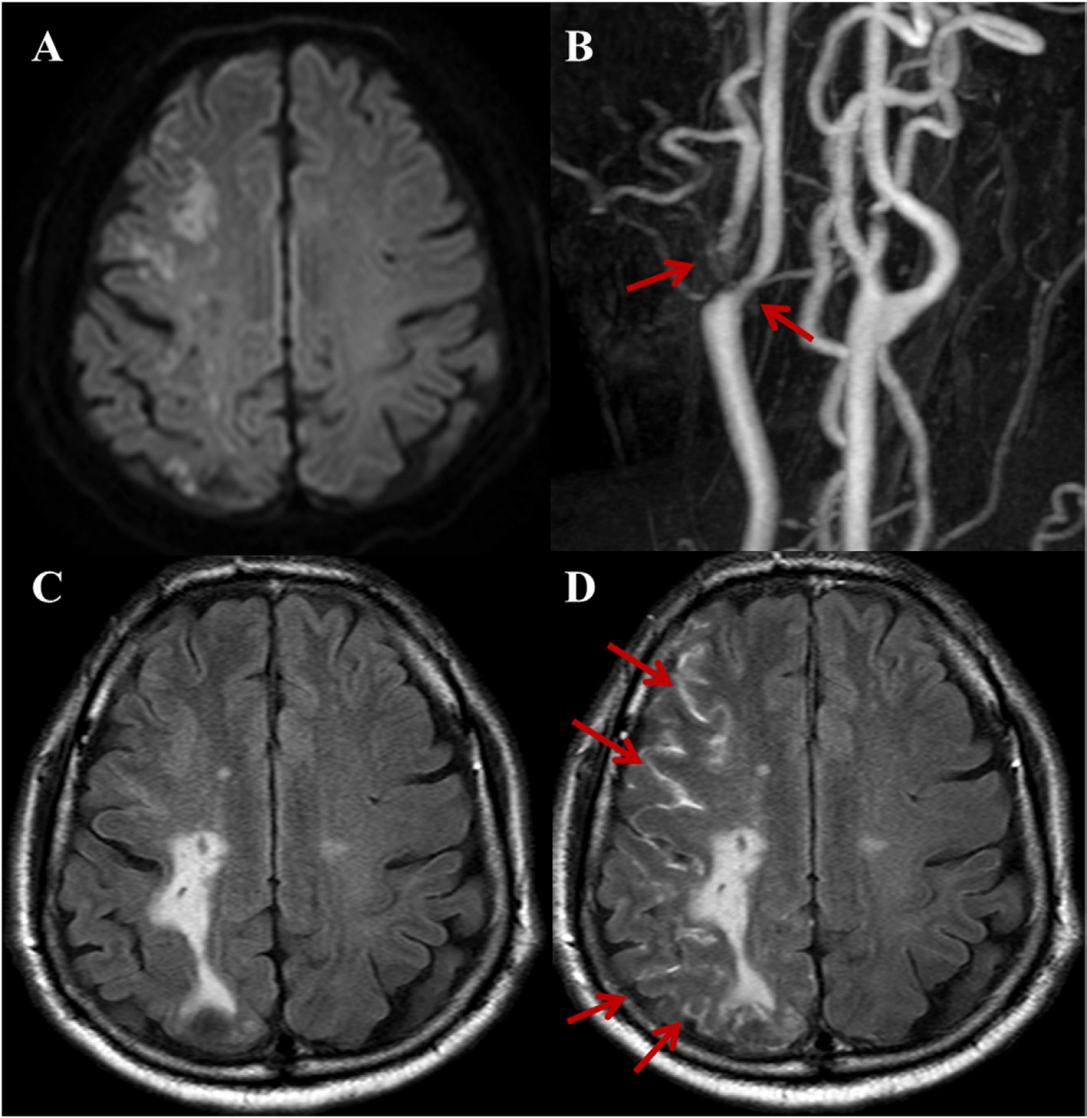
A 72-year-old male with a complaint of acute onset left arm weakness. Stroke due to large artery to artery atherosclerosis. (A) DWI shows acute border zone infarctions on the right cerebral hemisphere. (B) Three-dimensional time-of-flight MRA of extracranial arteries shows moderate-to-severe stenosis with plaque at right proximal internal carotid artery and ECA (arrows). Compared with non-contrast fluid-attenuated inversion recovery (FLAIR) (C), the post-contrast FLAIR (D) image shows diffuse sulcal enhancement (hyperintense acute reperfusion marker sign) along the right cerebral sulci (arrows).
